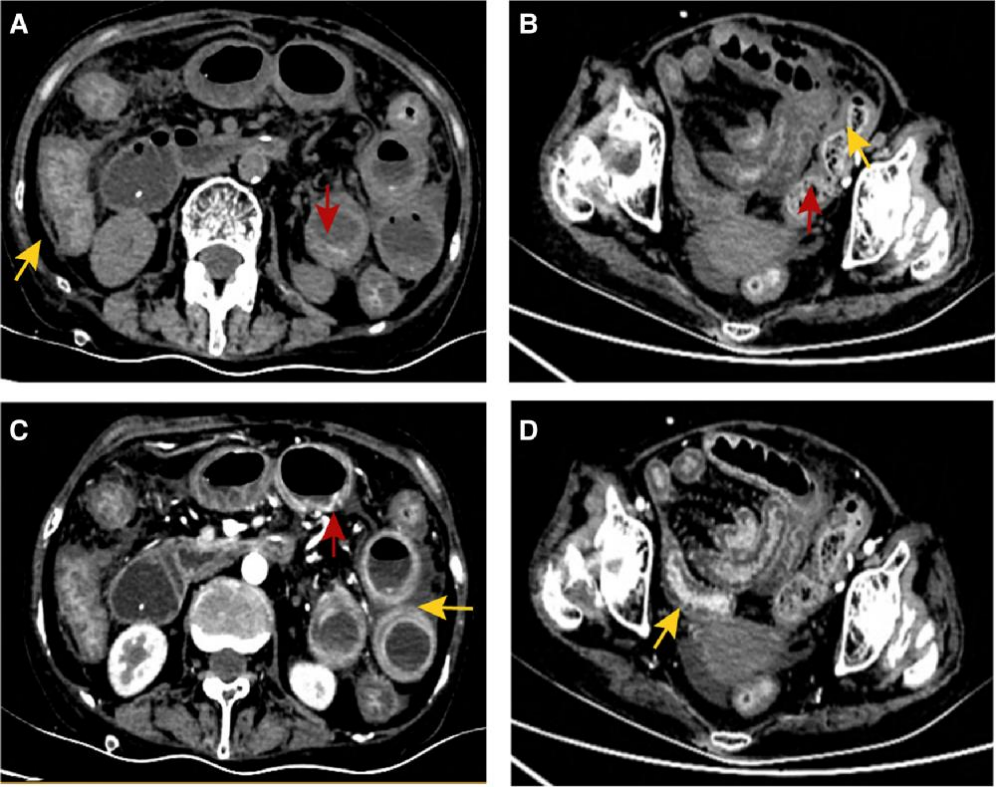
Representative image of abdominal computed tomography: *A* and *B*, scan of abdomen; *C* and *D*, contrast-enhanced scan of abdomen. A thickened bowel wall and the edema of mucosa are indicated (yellow arrows). Linear and annular high-density shadow on mucosal surface indicates mucosal hyperemia (red arrows). Meanwhile, blurred fat gaps around the bowel wall suggest effusion in the abdominal cavity.
